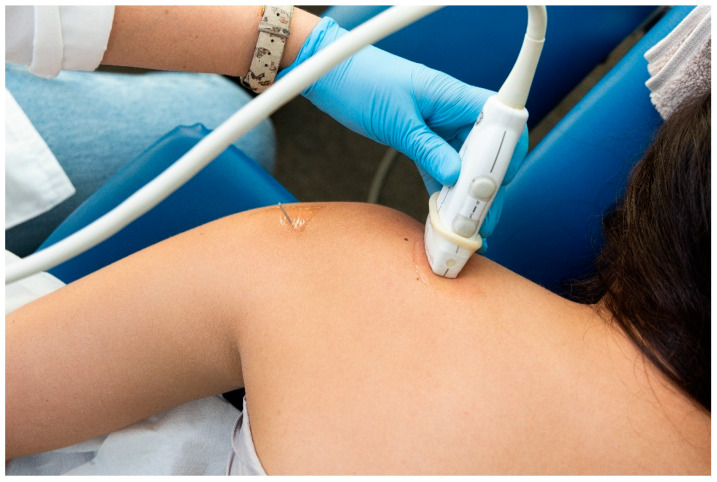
Placement of the ultrasound probe for identification of the suprascapular nerve.
